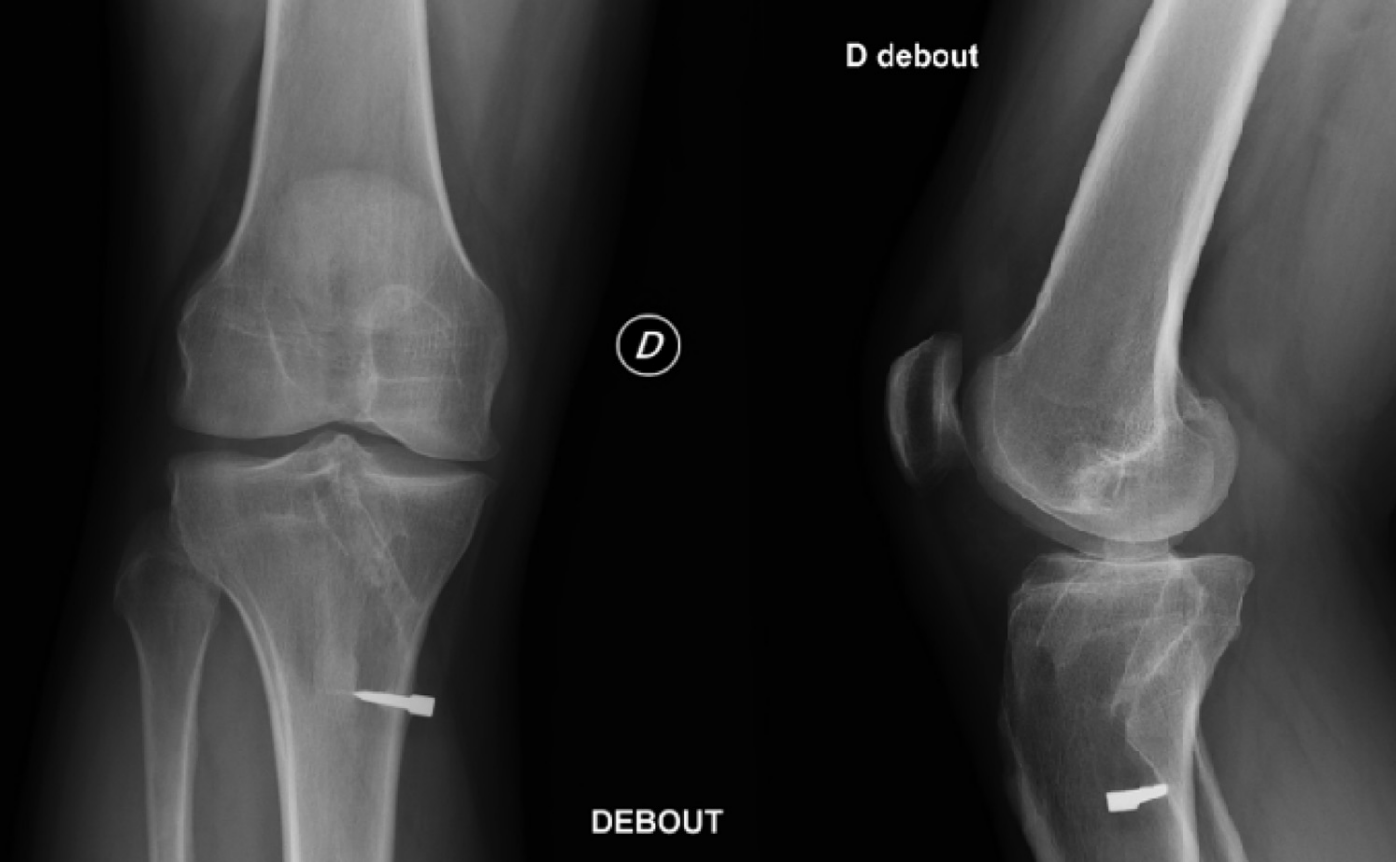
Preoperative radiographs of right (D) knee. The patient underwent prior anterior cruciate ligament and posterior cruciate ligament reconstruction surgery.
